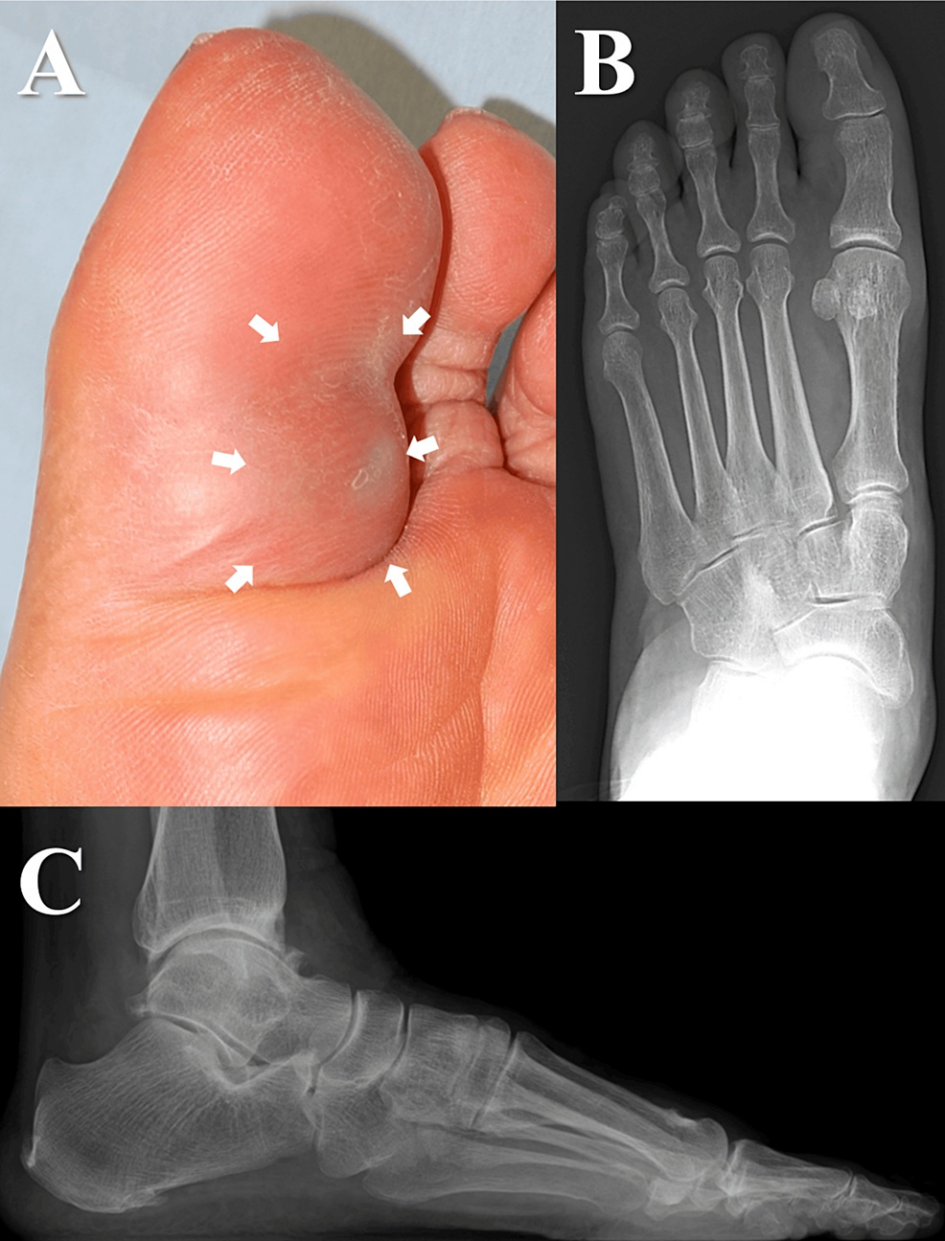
Preoperative clinical photograph and radiographic findings (Case 1) (A) A tender, elastic, firm, and mobile swelling is identified on the plantar aspect of the hallux. (B and C) Anteroposterior and lateral radiographs of the left foot show no remarkable changes.
